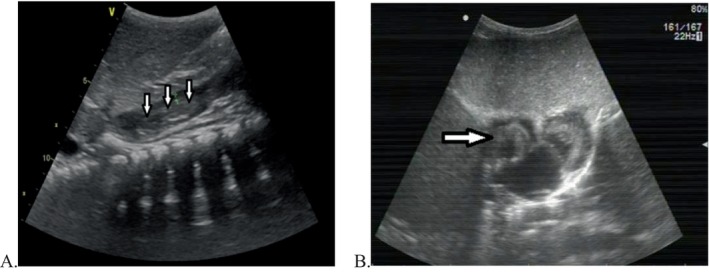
Preoperative transthoracic echocardiography (TTE) revealed a tumour thrombus in the inferior vena cava (IVC) (small arrows in A) and a tumour thrombus in the right atrium (large arrow in picture B).
